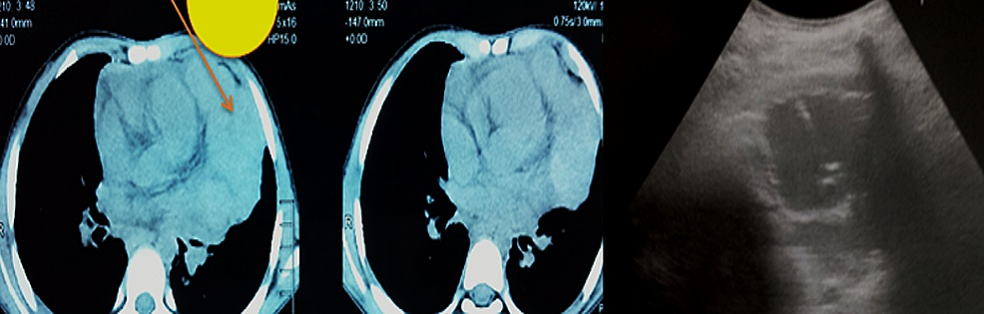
Plain CT scan of the chest showing a large anterior mediastinal mass along the pericardium. Ultrasound-guided accurate needle placement was performed for transthoracic mass biopsy.
